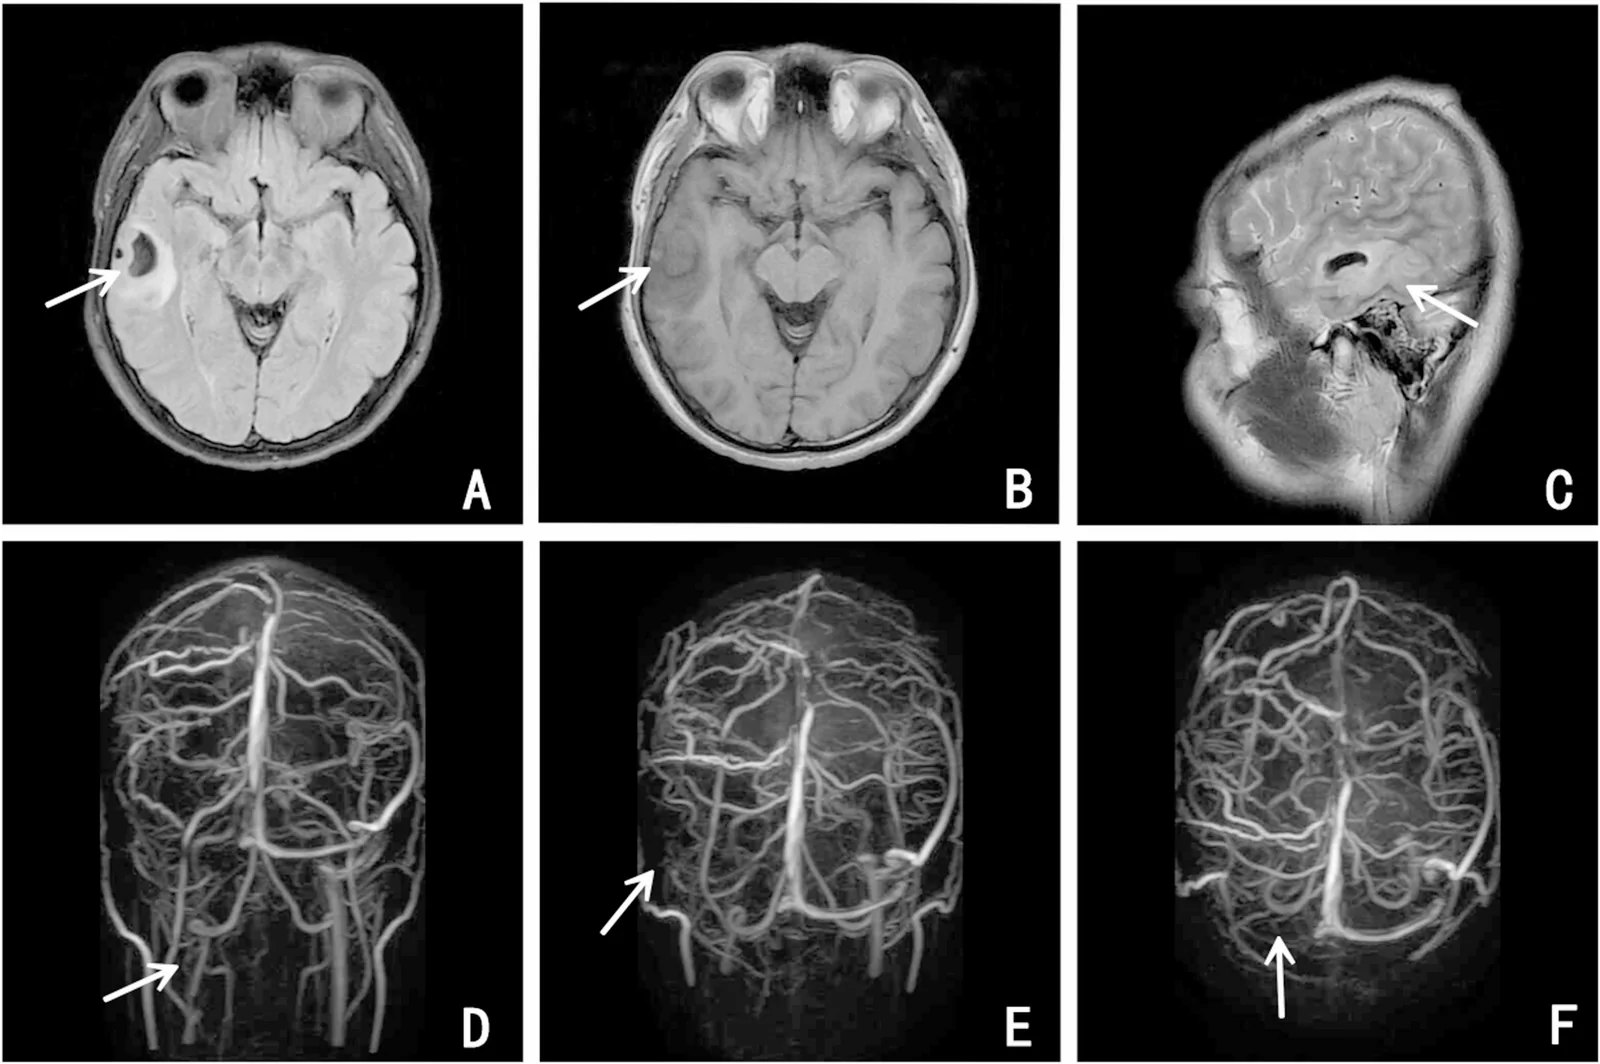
Head MRV demonstrating CVST in a proband with hereditary PSD. Note: A: Axial T1-weighted image showing the right temporal lobe infarction (arrow). B: Axial T2-weighted FLAIR image showing right temporal lobe infarction (arrow). C: Axial diffusion-weighted image showing right frontal lobe infarction (arrow). D: Maximum intensity projection (MIP) angiography showing a partial filling defect in the right internal jugular vein (arrow). E: MIP angiography occlusion of the right sigmoid sinus (arrow). F: MIP angiography showing a filling defect in the right transverse sinus (arrow).
